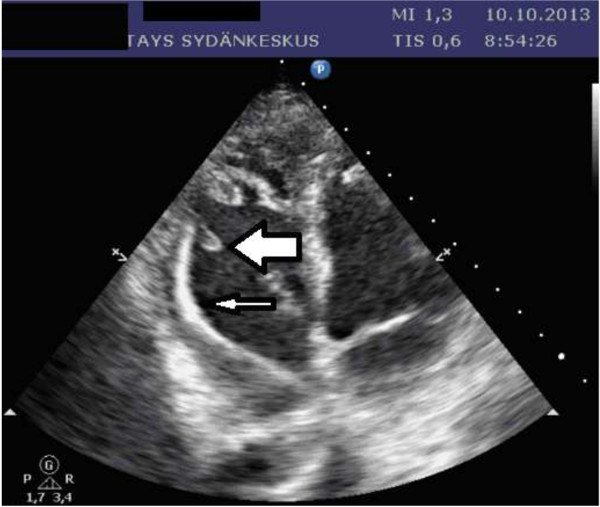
Transthoracic echocardiography examintion of the second patient. The slender arrow points to the ventricular pacemaker lead. The bold arrow points to the fluttering vegetation mass.
